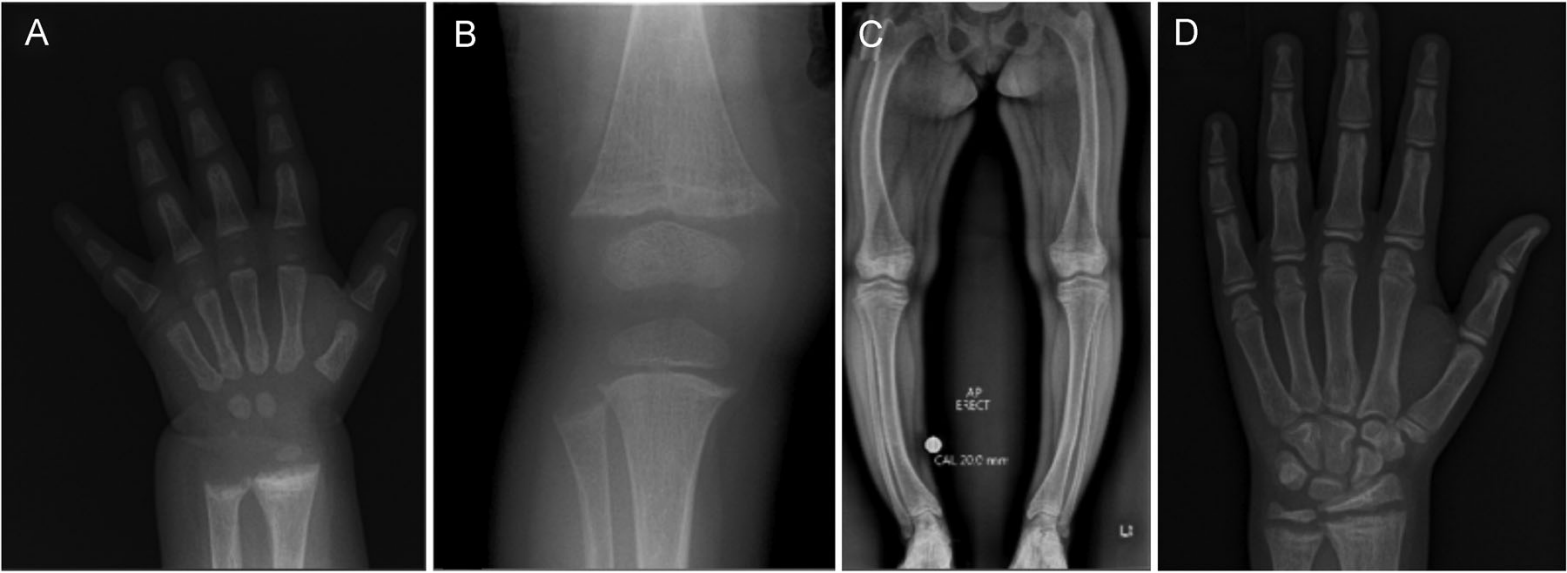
A now 14‐year‐old female with XLH (PHEX:c.[151C>T];[=] p.[Gln51*]) diagnosed at 7 months of age. (*A*) Radiograph of left hand and wrist at diagnosis with rachitic changes of distal radius and ulna and lacey appearance of bone. (*B*) Radiograph of right knee at 18 months old while treated with phosphate and calcitriol. There is fraying and splaying at the metaphyses and early cupping noted at the distal femur as well as the proximal tibia and fibula. (*C*) At 14 years old, she was managed with phosphate and calcitriol. She had short stature, normal ALP, and mild elevation in PTH. Symptoms included persistent ankle pain and waddling gait. There was also lateral bowing of both femora and tibias with widening of the proximal tibial growth plate. (*D*) Left hand radiograph at age 14 years showing widening of the proximal radius and ulna growth plates with evidence of rachitic changes.
